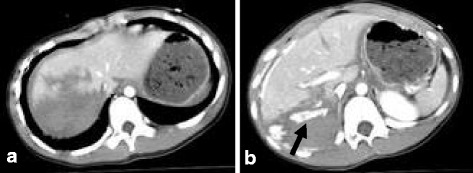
a, b Axial view of an enhanced abdominal computed tomography (CT) scan shows the presence of active extravasation (*arrow*), large devascularization of the right hepatic lobe, and hemoperitoneum
